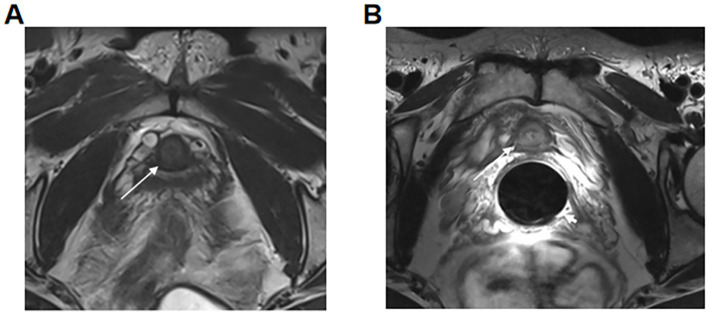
T2-weighted imaging (T2WI) of the same patient. (A) Abdominal coil image. The arrow indicates the urethra. (B) Vaginal coil image. The long arrow indicates the urethra, and the short arrow points to the coil placed within the vagina.
